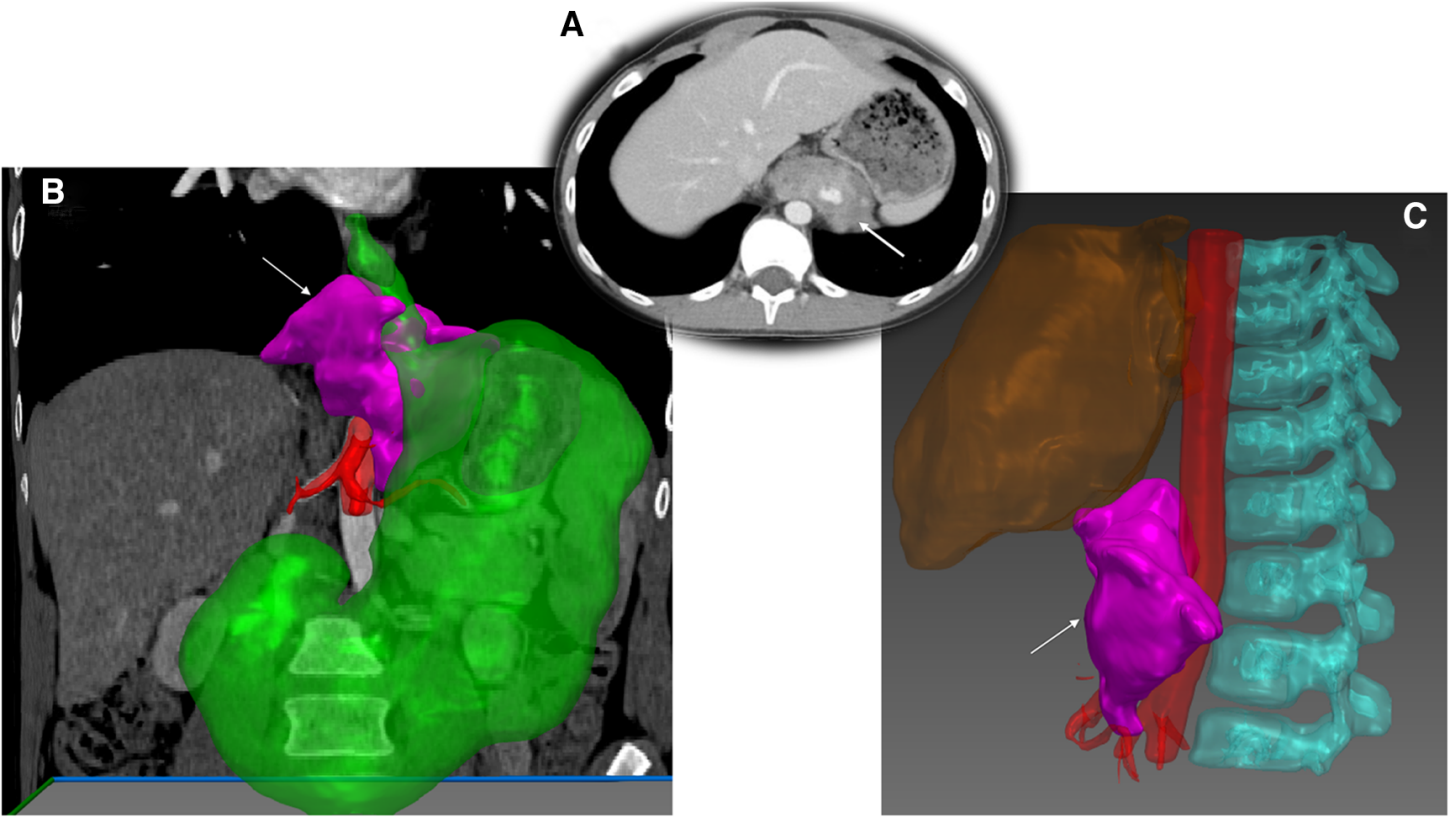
Abdominal inflammatory myofibroblastic tumor and 3D modelling. (A) Computed tomography acquisition of a retroperitoneal mass in a 12-year-old boy. (B) Segmentation of the tumor (arrow) using Medical Imaging Interaction Toolkit (MITK°-free open source software-German Cancer Research-Germany) showing close contact with abdominal aorta (C).
